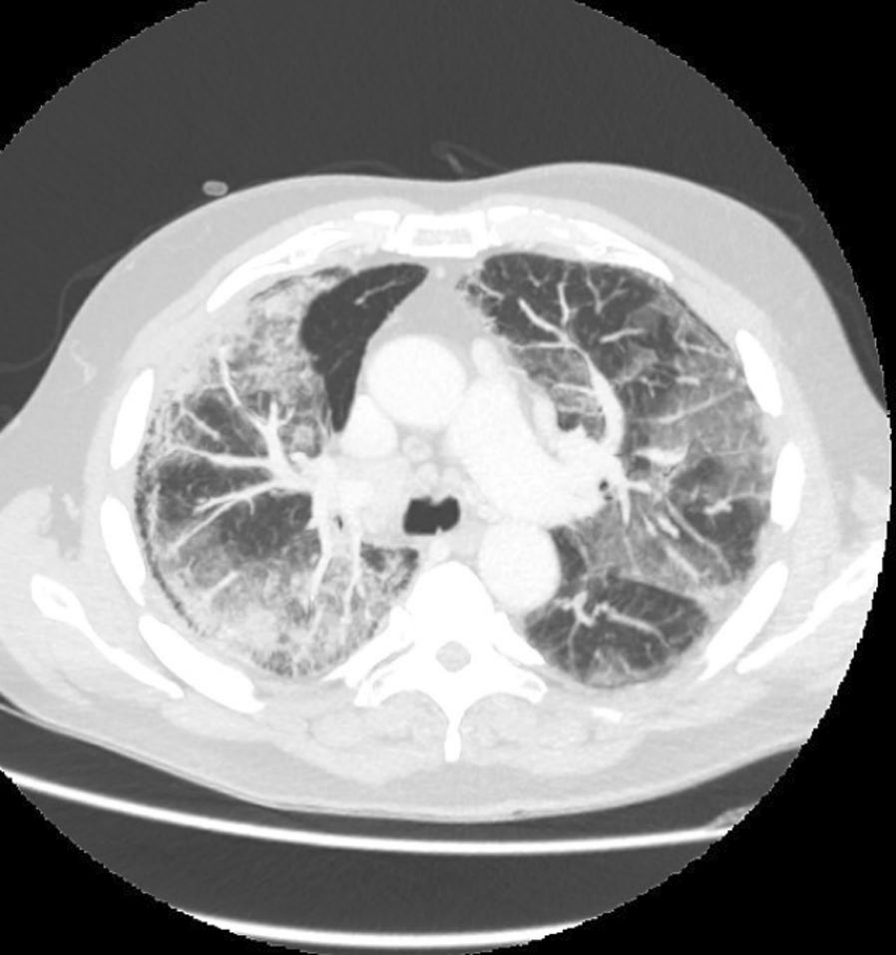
Case 2—CT chest upper lobes with deterioration of pneumonitis despite treatment
